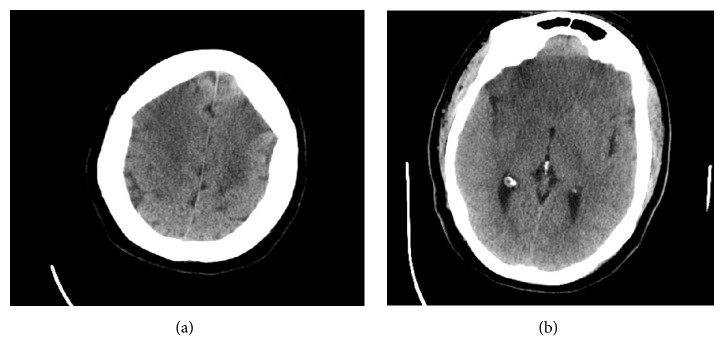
Axial CT images demonstrating bilateral large areas of hypoattenuation consistent with acute MCA and ACA infarcts.
